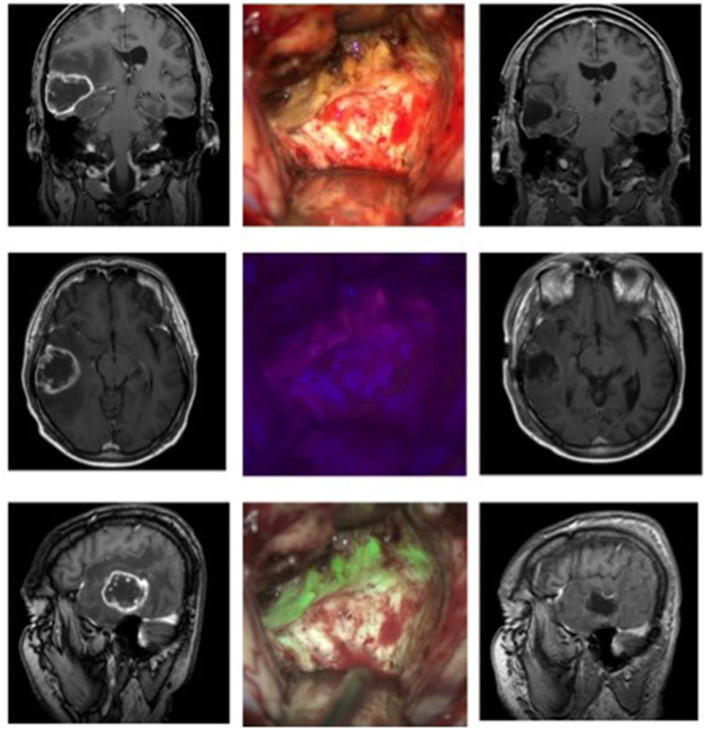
Glioblastoma in a 53-year-old patient. The left column shows the MRI images (T1 weighted with contrast, from top to bottom: coronary, axial, sagittal) before the operation, the right column the postoperative MRI. In the middle column, the top picture shows the surgical field under white light, below it under blue light, and at the bottom the fusion of both images in the MFL-mode.
